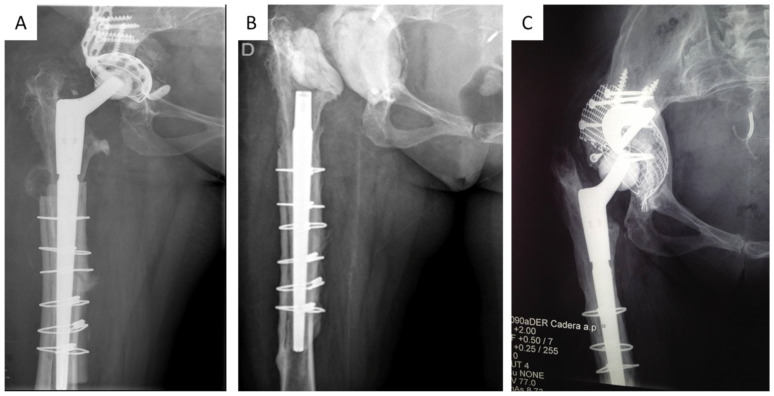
(A), Preoperative radiograph before the first stage in a patient with septic loosening of acetabular component; (B), radiograph with a non-articulated spacer; (C), radiograph at 9-year follow-up after the second stage with bone impaction grafting and trabecular metal augments (BIG-TMT).
